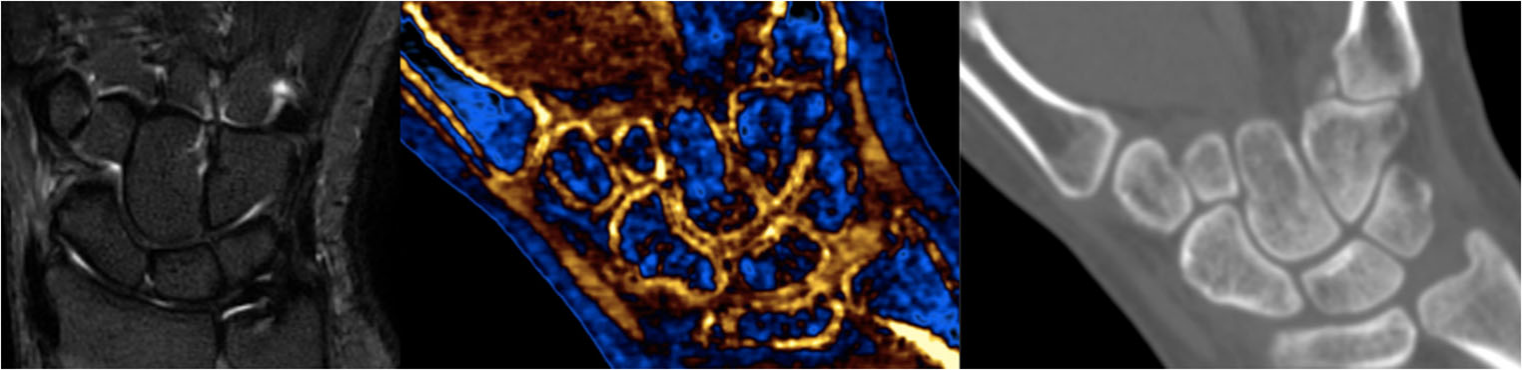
Normal scaphoid. No acute scaphoid bone marrow oedema on the STIR sequence of the MRI (left), DECT with calcium/water filter (middle) and no fracture on the CT component (right)
